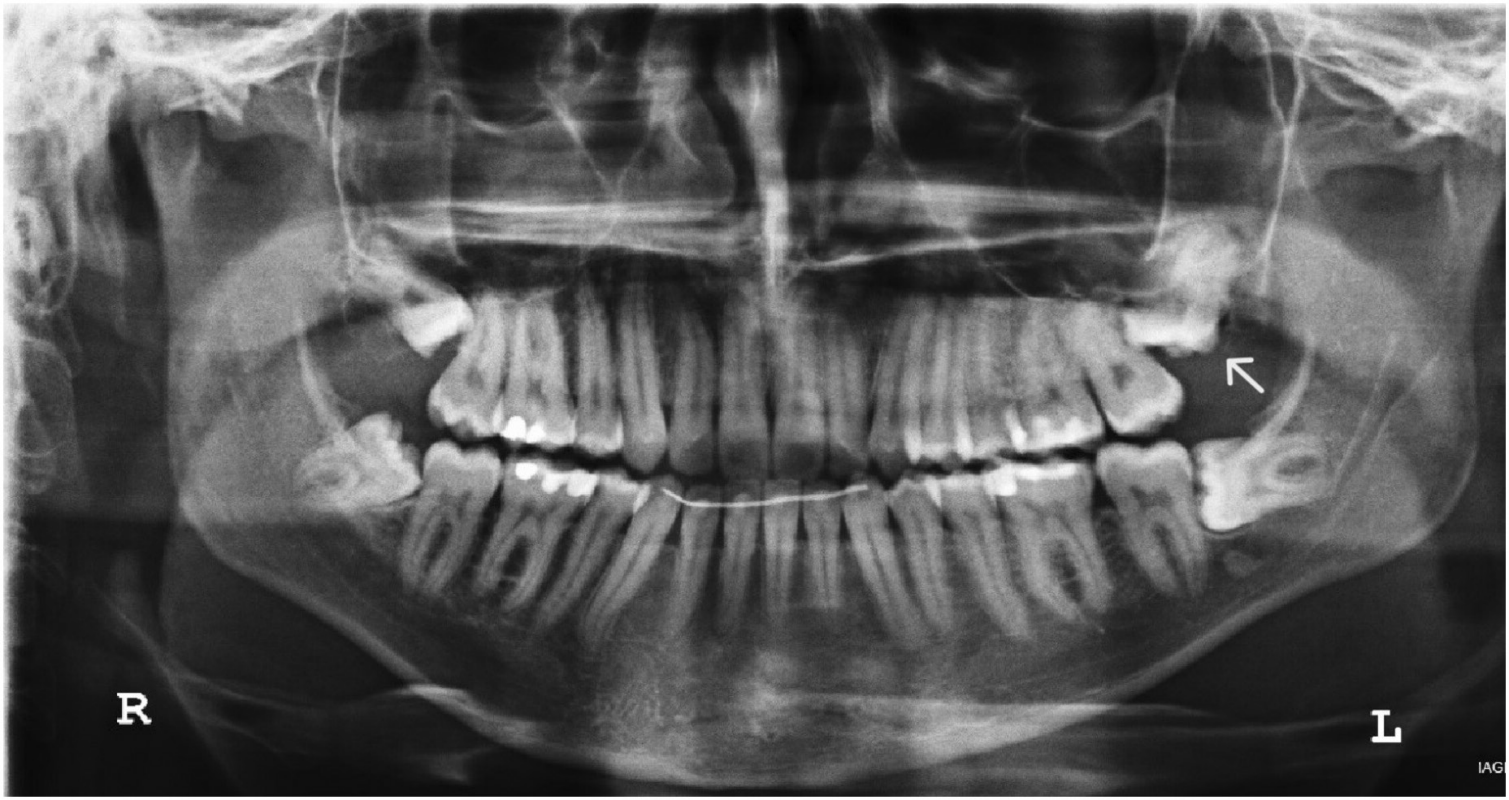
Initial panoramic radiograph. White arrow shows the impacted third molar with small changes in size and shape.
